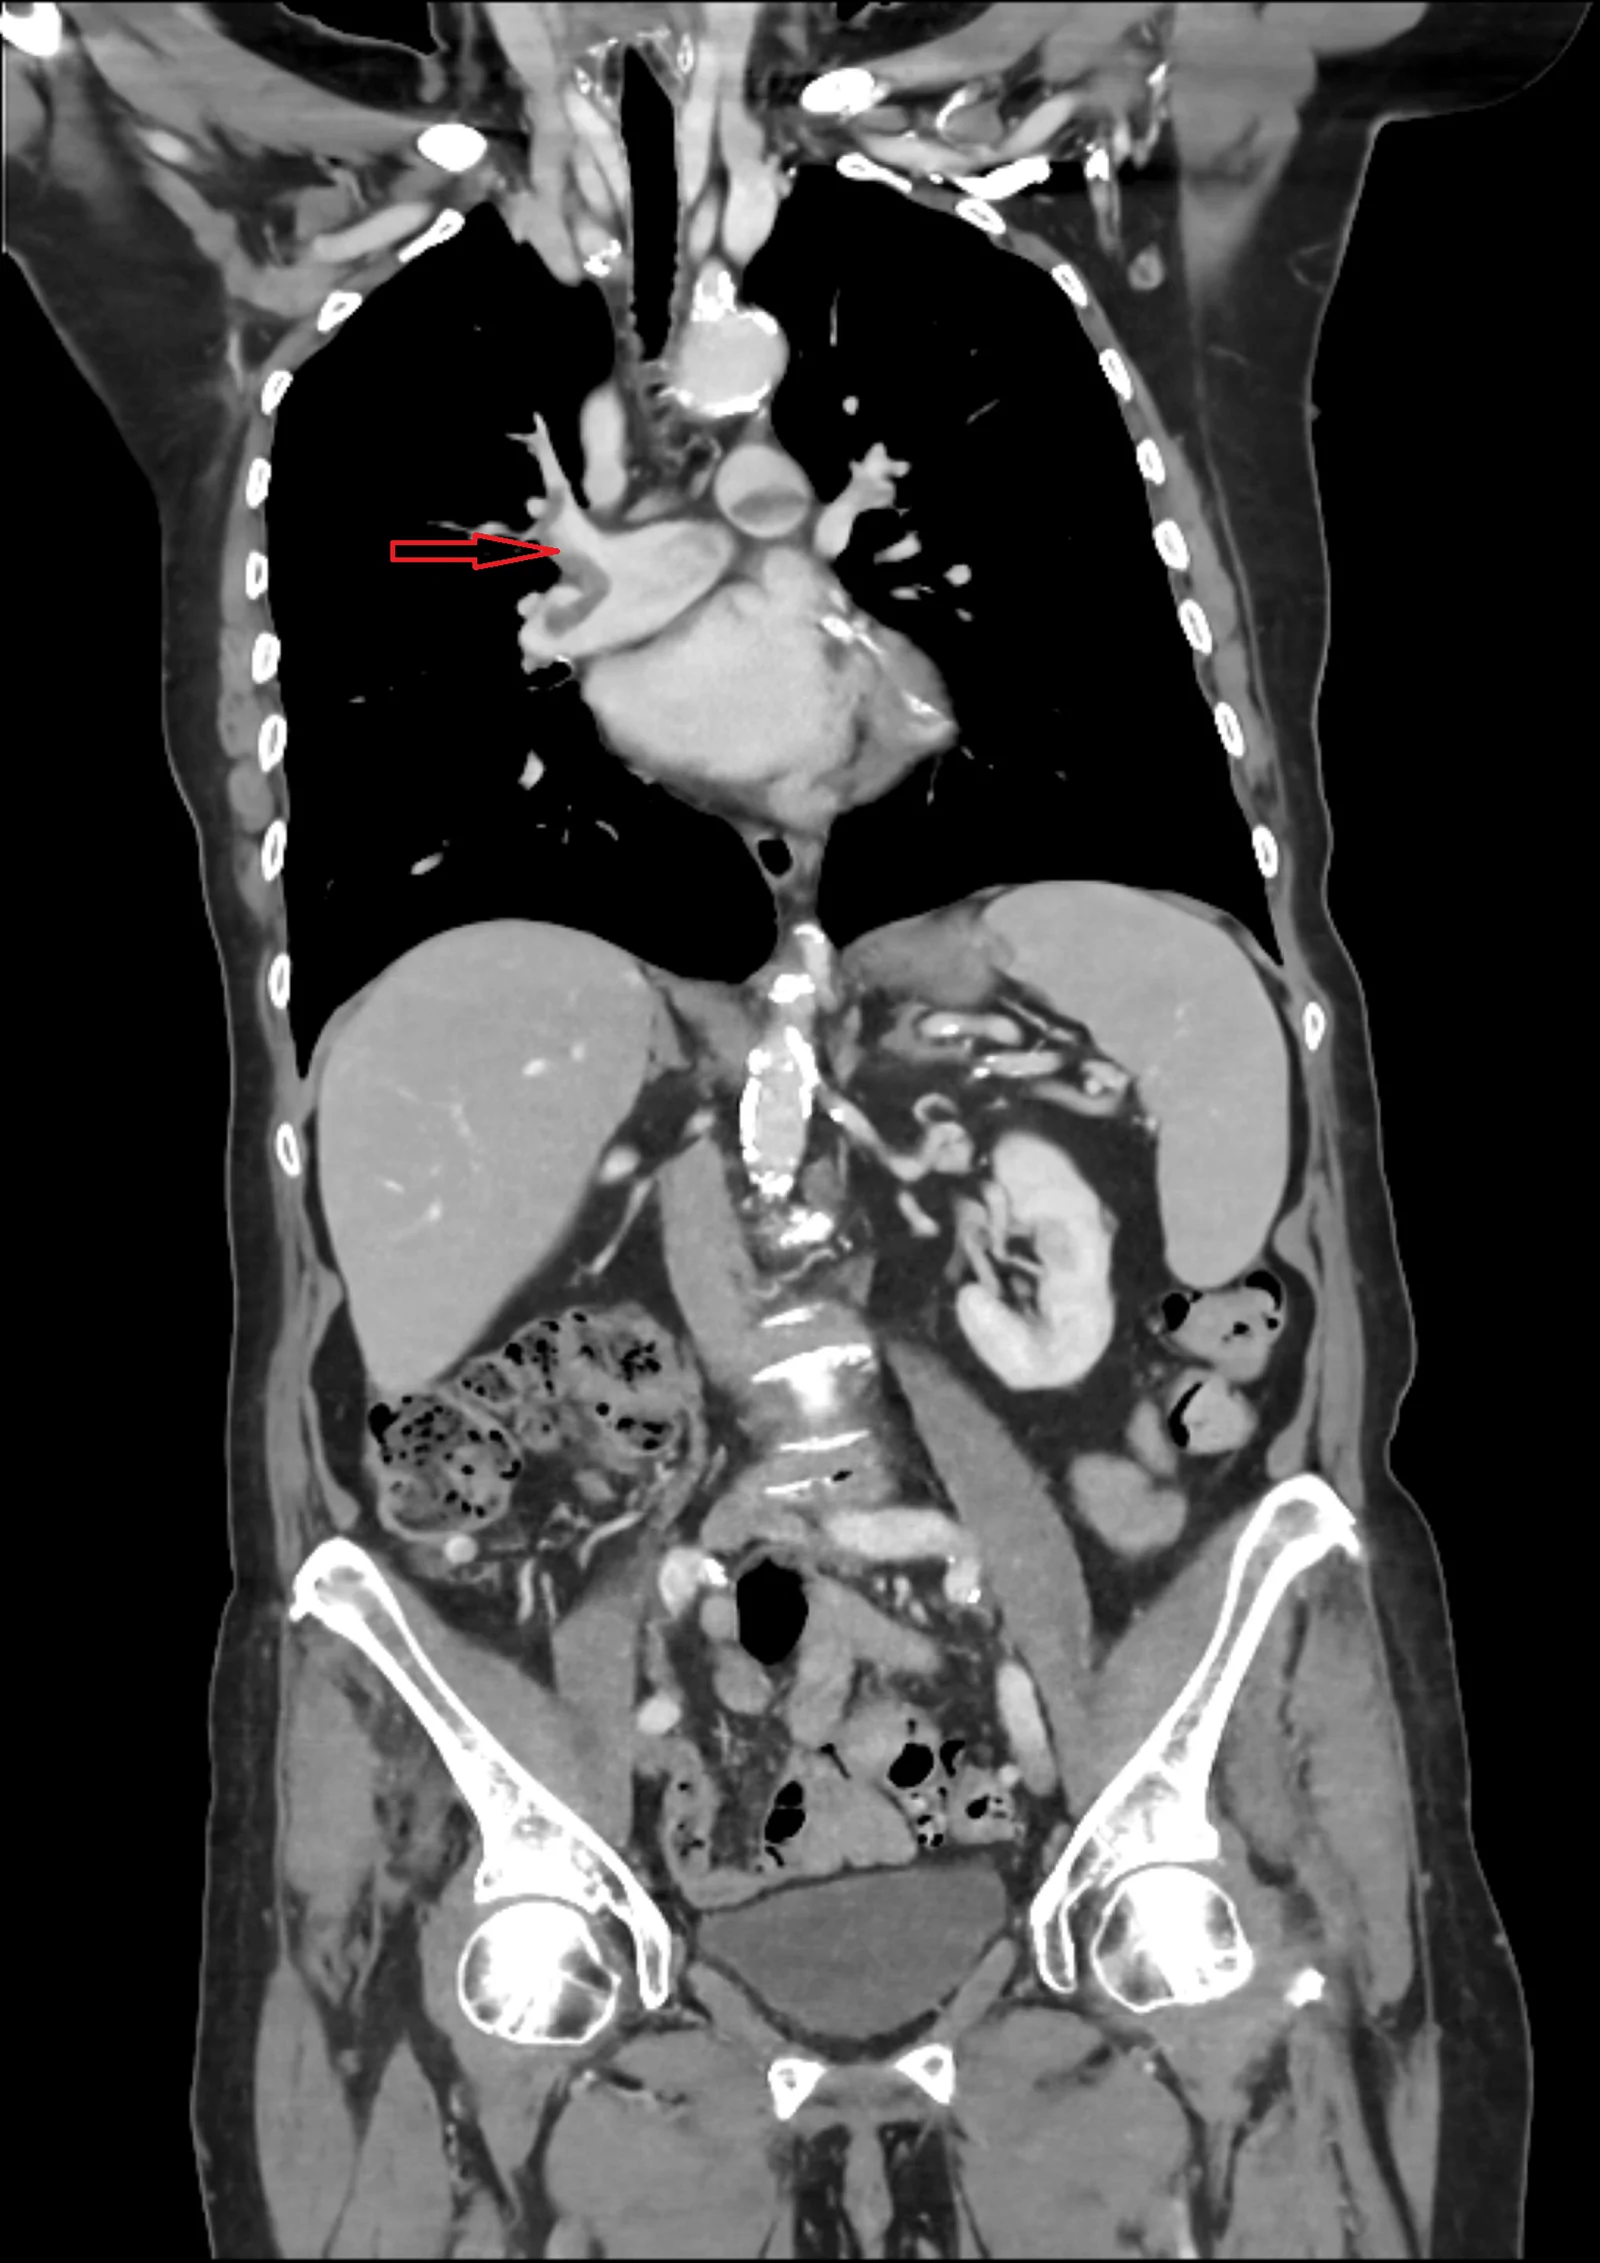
CT thorax, abdomen and pelvis with contrast, with the red arrow pointing towards large pulmonary embolism.
